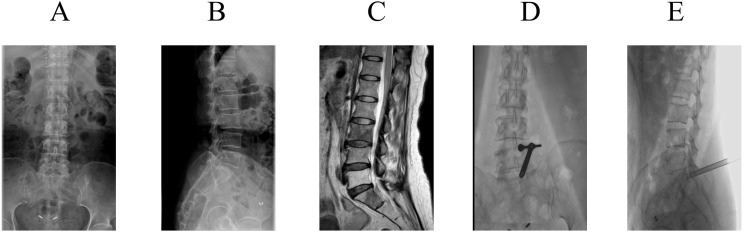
Preoperative and intraoperative imaging of the patient. (A) Anteroposterior (AP) lumbar spine digital radiograph. (B) Lateral lumbar spine digital radiograph. (C) Preoperative magnetic resonance imaging (MRI) of the lumbar spine showing bone metastasis. (D) Anteroposterior fluoroscopic image demonstrating the positioning of the endoscopic working cannula. (E) Lateral fluoroscopic image demonstrating the positioning of the endoscopic working cannula.
